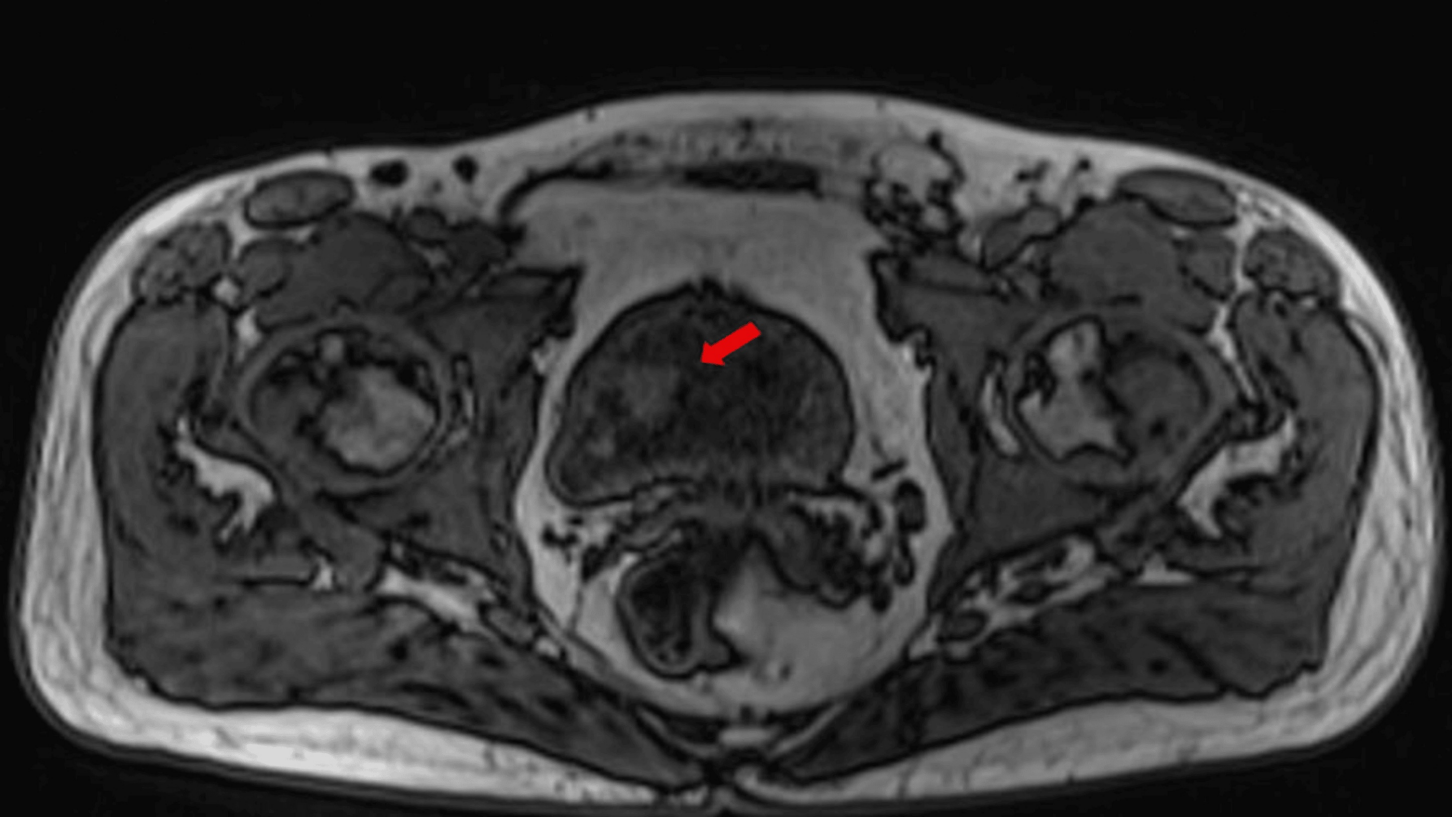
Pelvic MRI showing nodular thickenings of the right bladder wall. Plane: Axial (transverse), passing through the pelvic region; Sequence: Fast Spin Echo
